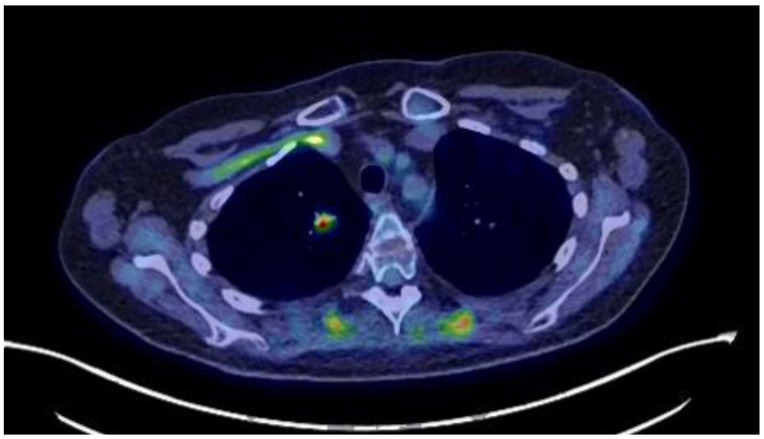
^18^F FDG PET/CT showing a hypermetabolic region in the right lung.
